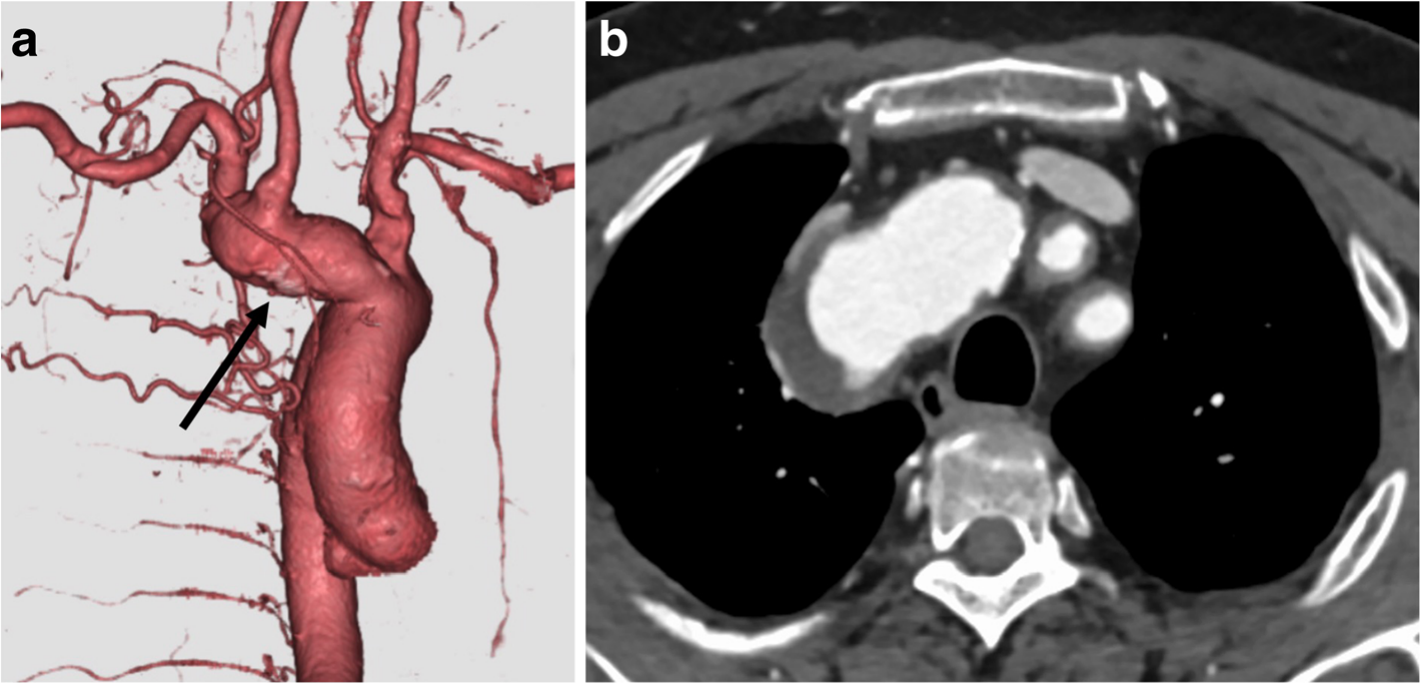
a Preoperative computed tomography showing brachiocephalic artery aneurysm (black arrow) measuring 32 mm in diameter with thickened aortic wall. b Preoperative computed tomography showing severe atherosclerotic changes in the aortic arch and orifice of the arch vessels
